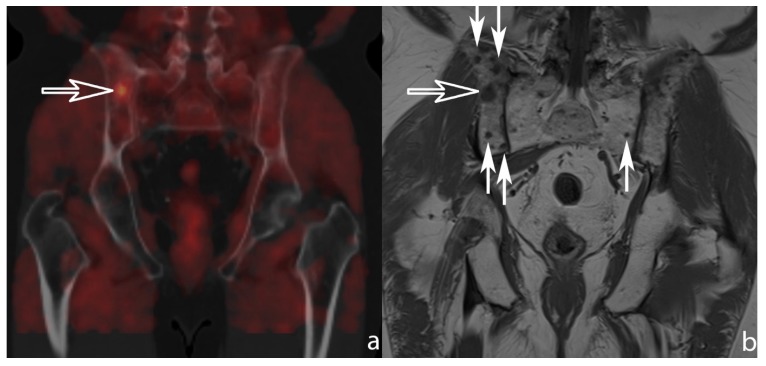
(a) Coronally reformatted fused choline positron emission tomography/computed tomography (PET/CT) image (left) and (b) 5 mm coronal T1-weighted magnetic resonance (MR) image of the sacro-iliac joints. In this patient three suspected bone metastases were reported on the PET and CT images making this patient eligible for metastasis-directed therapy. One of the lesions was located posteriorly in the right iliac bone (open arrow). On MRI, however, multiple T1-hypo-intense nodules (small arrows) can be observed indicative of diffuse metastatic disease warranting systemic treatment.
